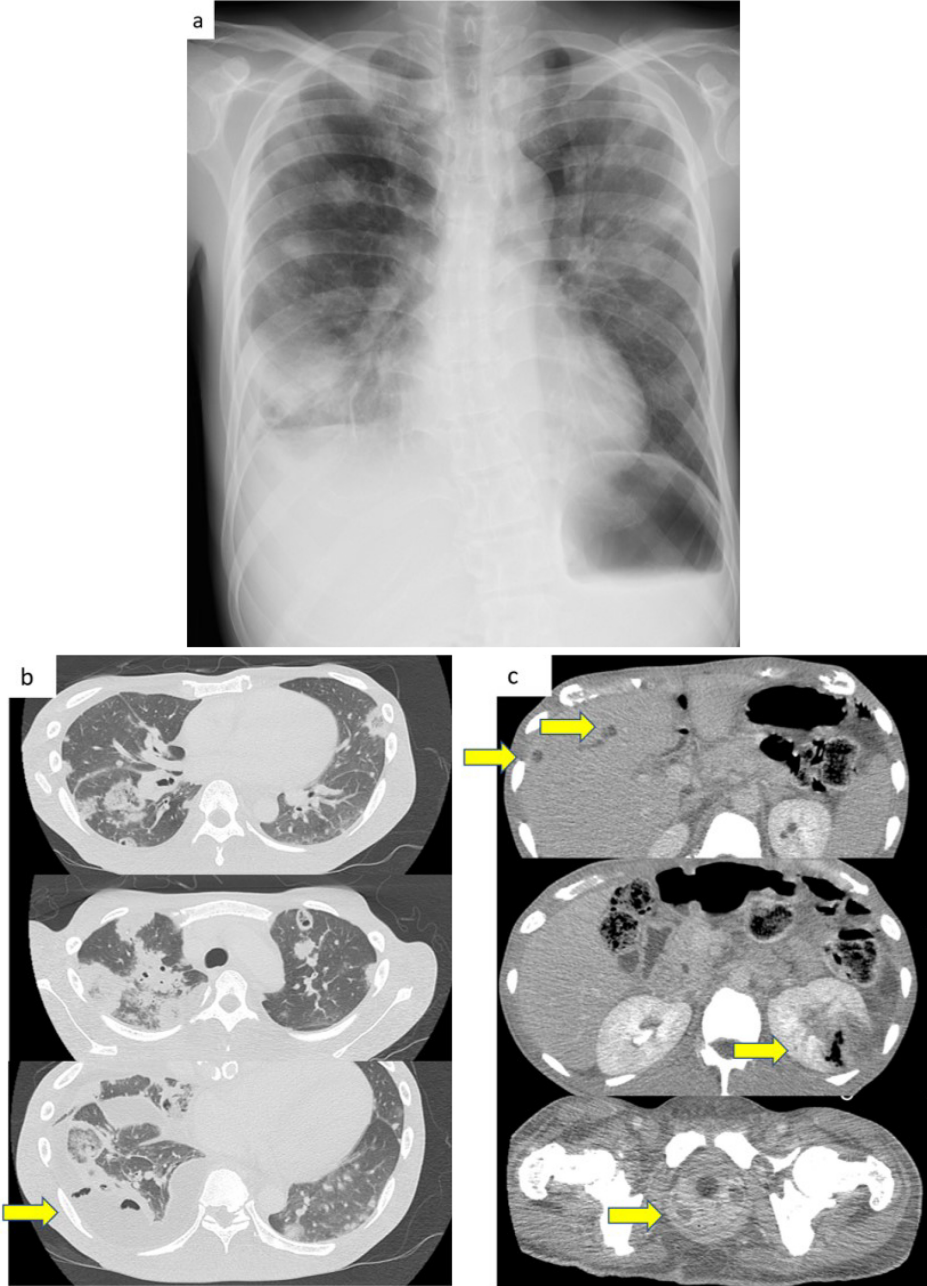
Imaging findings Figure 1-a. Chest radiograph at presentation shows multiple infiltrative shadows involving both lung fields Figure 1-b. Chest computed tomography performed on day 2 of hospitalization shows right empyema (arrow) Figure 1-c. Contrast-enhanced abdominal computed tomography shows liver abscess, emphysematous pyelonephritis, and a prostate abscess (arrow).
